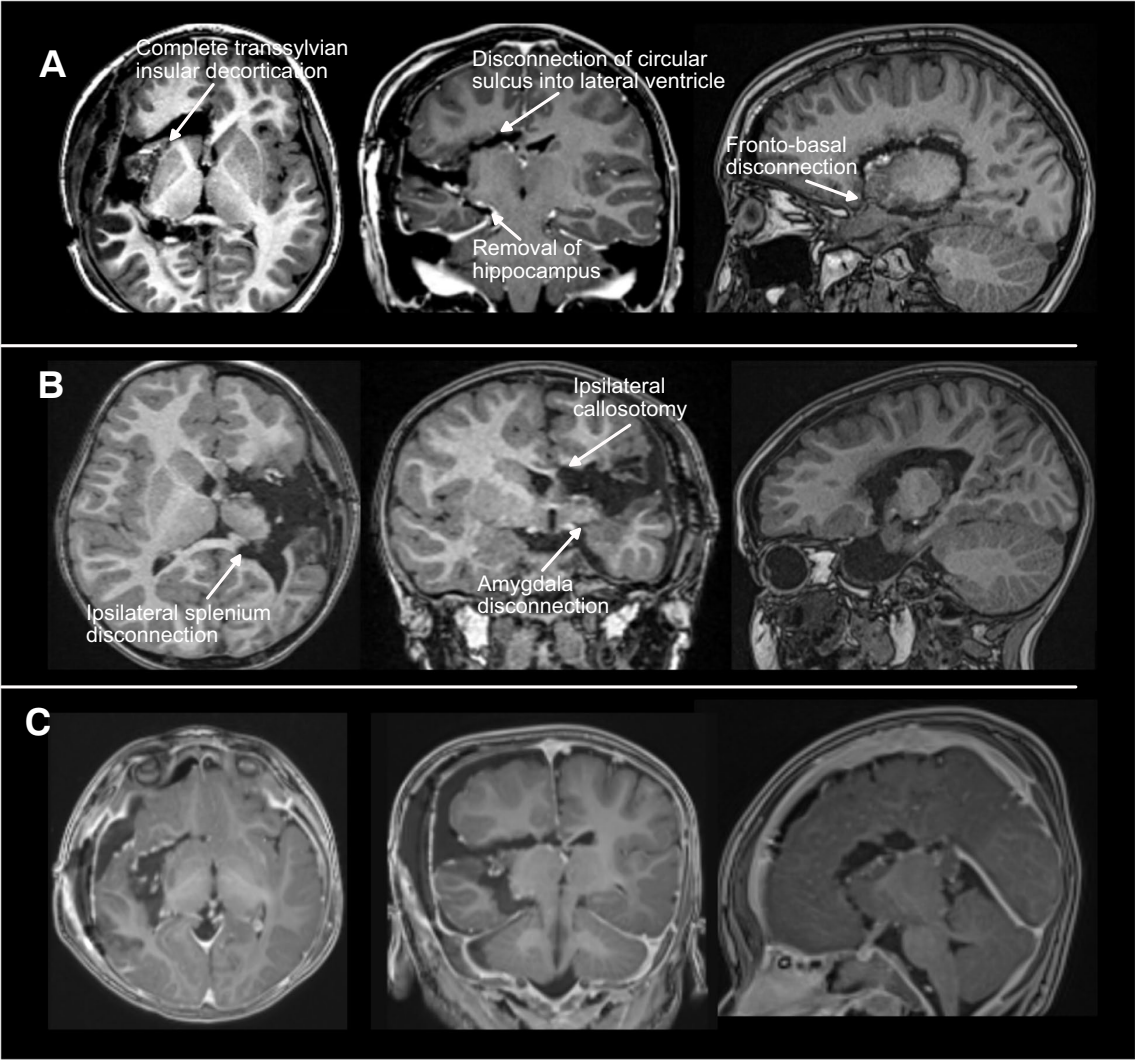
Representative post-operative MRIs following modified transsylvian hemispherotomy. T1-weighted axial, coronal, and sagittal images are displayed. A 11-year-old with Rasmussen’s encephalitis. B 4-year-old with perinatal L MCA stroke. C 15-month-old with hemispheric dysplasia. Radiographic correlates of several key disconnection steps are annotated
